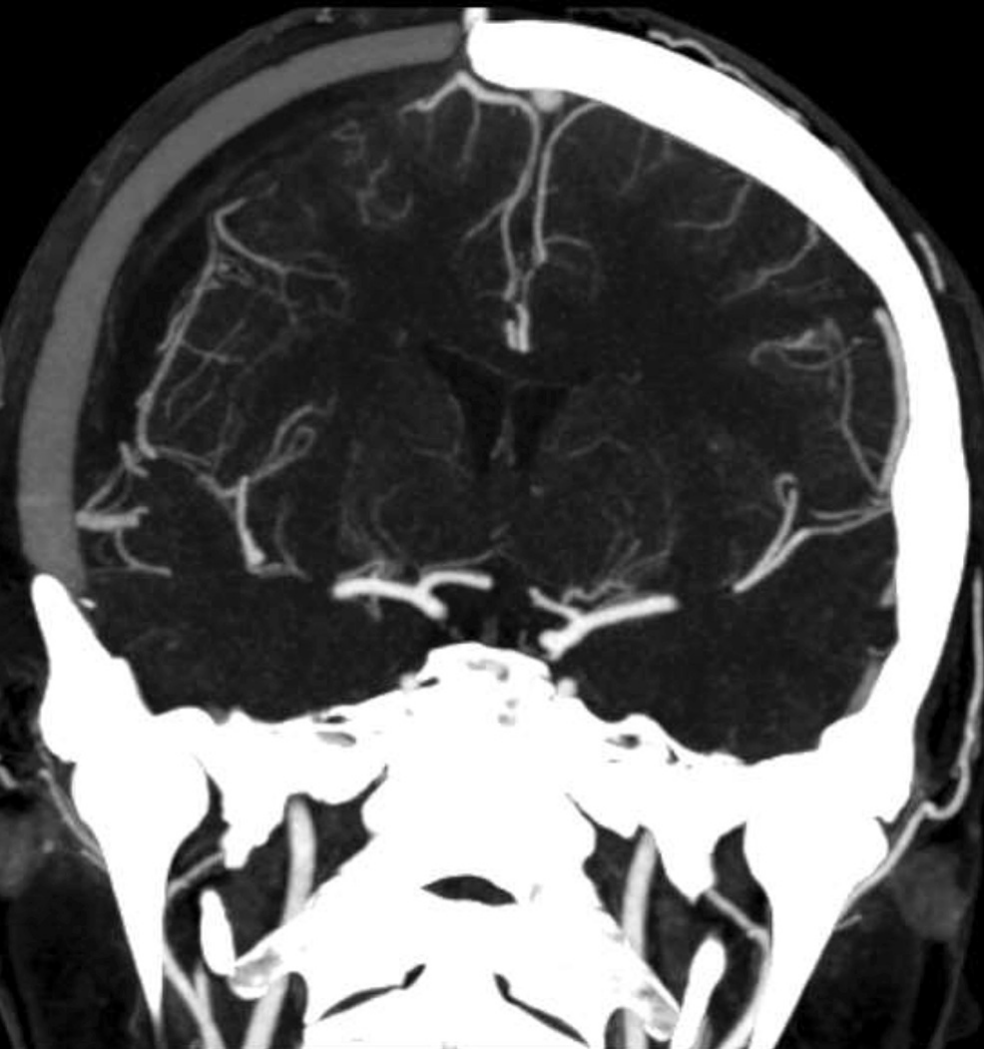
Post-operative day 1 computed tomography angiogram showing no residual brain arteriovenous malformation
